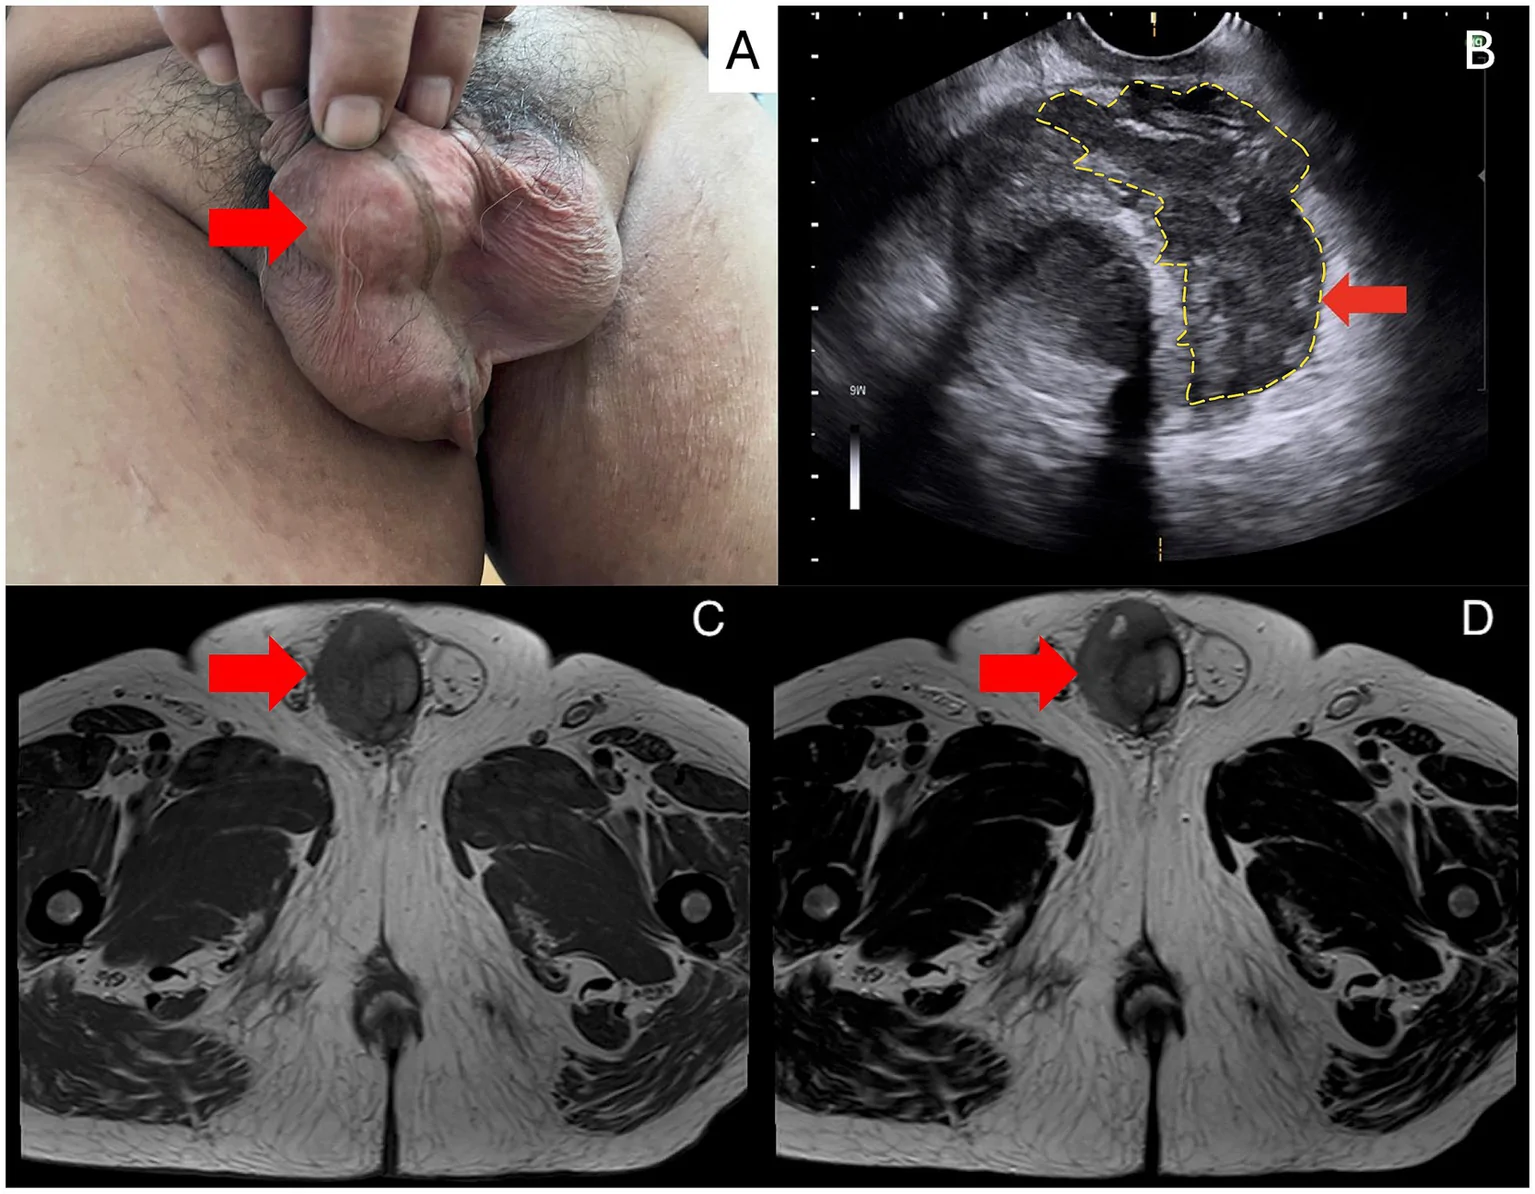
(A) Clinical photograph showing diffuse penile enlargement with a prominent mass predominantly involving the right side of the penile shaft (arrow), corresponding to the ultrasound-guided biopsy site. (B) Penile ultrasonography demonstrates a heterogeneous mass involving the corpora cavernosa and confined within the tunica albuginea, without definite invasion of the urethra. (C,D) Pelvic MRI shows an 8 × 4.6 cm mass involving the right corpus cavernosum and tunica albuginea, hypointense on T1- and T2-weighted images with restricted diffusion, suspicious for malignancy. No enlarged pelvic lymph nodes are seen.
